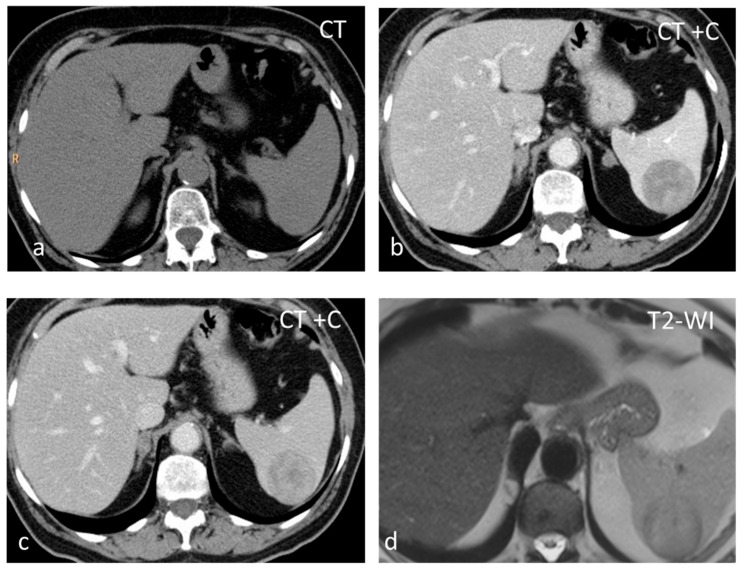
Large solid lesion, in keeping with an inflammatory myofibroblastic tumor, slightly hypodense in the non-contrast CT scan (a), depicting slightly heterogenous/patchy gradual enhancement pattern post-contrast enhancement (b,c). The lesion appears iso– to hypointense on T2-weighted MRI, more conspicuous than in the non-contrast CT scan (d).
